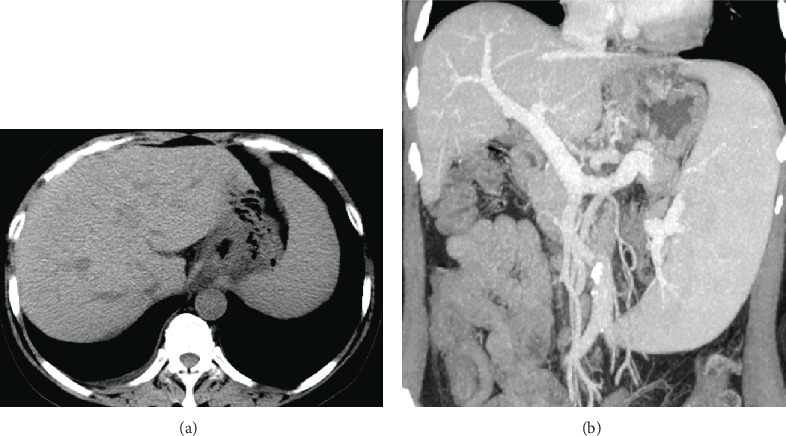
The abdominal enhanced CT showed no signs of cirrhosis. The splenic vein was significantly widened, and the spleen was enlarged.
